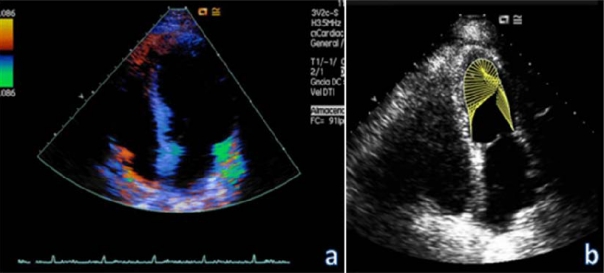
Left ventricular segmental contractility alterations. Evaluation by quantitative tecniques based on tissue Doppler image (a), or the velocity vector analysis (b).
